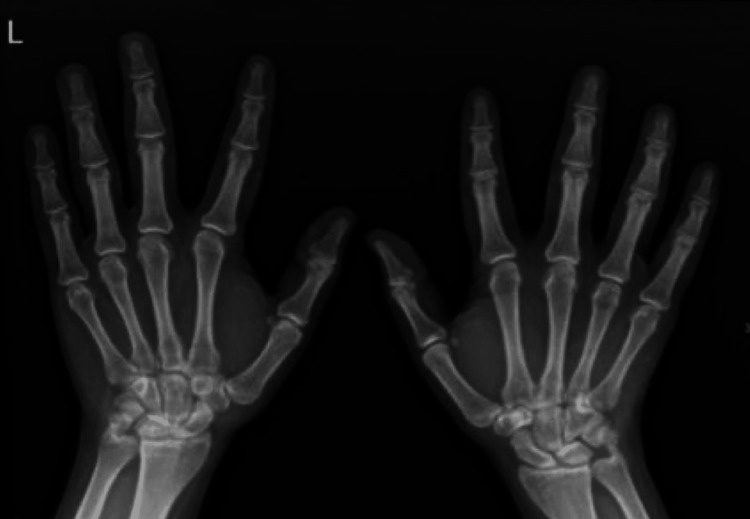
Posteroanterior X-ray view of the left hand.
